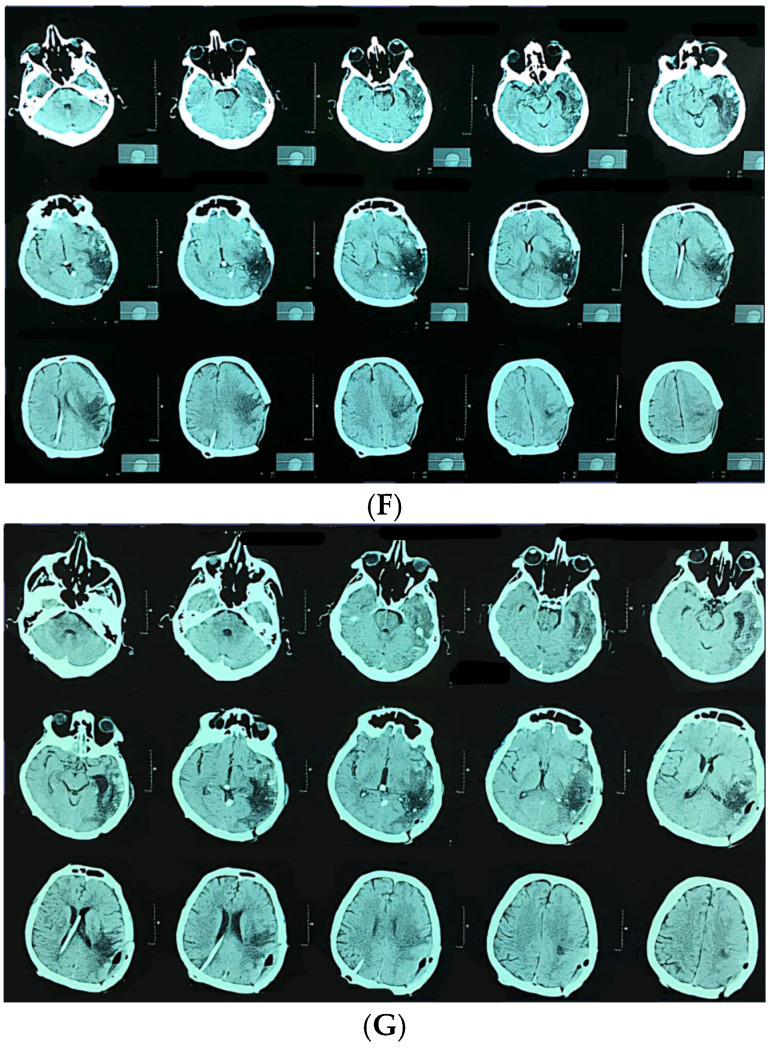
A 58-year-old man presented with sudden onset of headache and vomiting. Brain CT showing initial findings of intraventricular and intracerebral hemorrhage (A). Two hours after admission he became unresponsive, GCS 6; urgent CT showed hydrocephalus (B). Brain CT after the VP shunt operation (C). CT angiography was performed, confirming the diagnosis (D). Microsurgical resection of the AVM was performed, and after the operation the patient was unresponsive with hemiplegia. Control brain CT showed cerebral infarction with intracerebral hemorrhage (E). CT after the reoperation (F) And 6 months after the operation and cranioplasty surgery (G).
